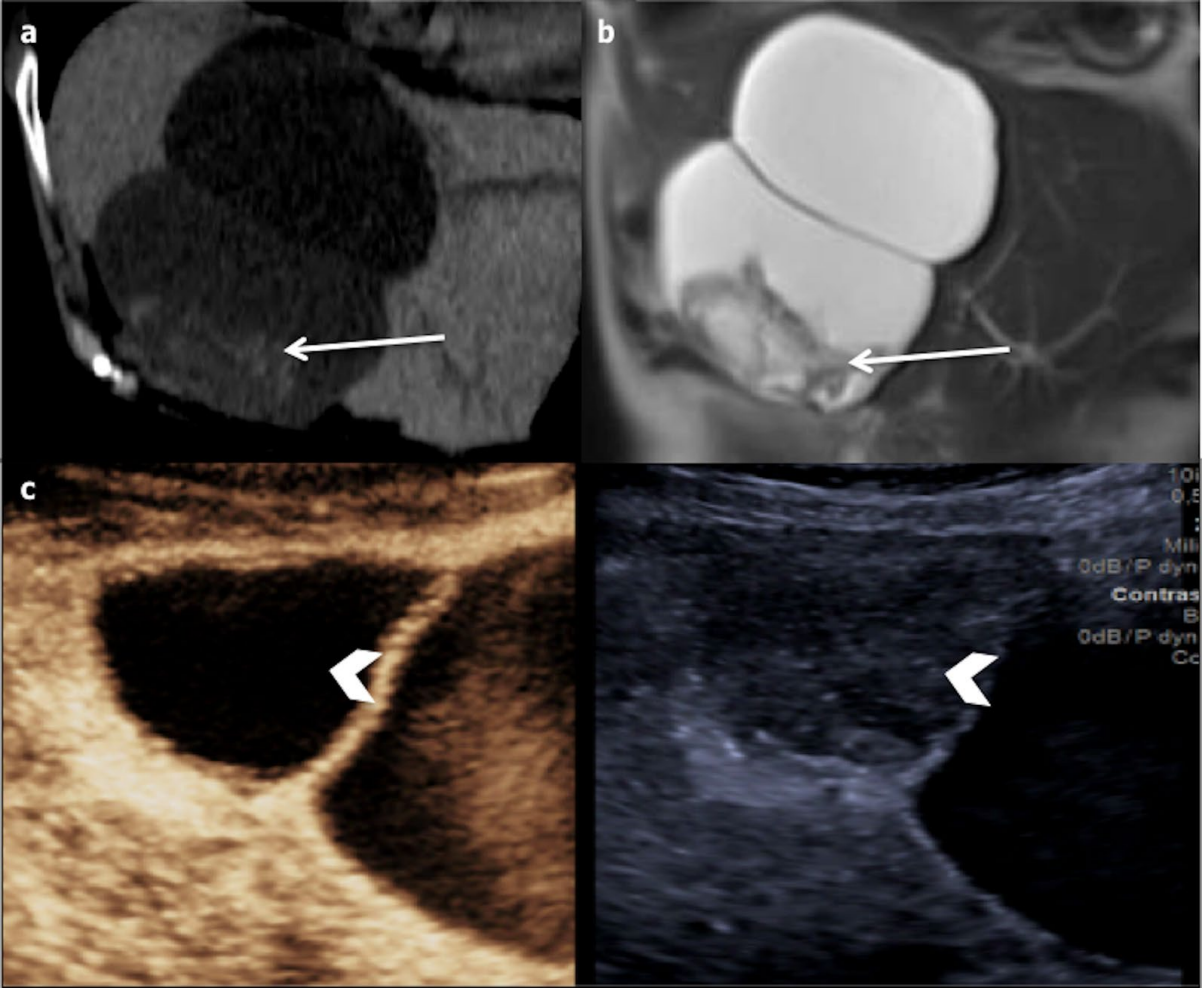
Bilobulated haemorrhagic hepatic cyst in a 73-year-old female. a Coronal non-enhanced computed tomography shows a bilobulated cyst with focal hyperattenuation in the inferior part (arrow); b coronal T2-weighted magnetic resonance imaging shows heterogeneous hypointensity inside the inferior part of the cyst (arrow). c Contrast-enhanced ultrasonography confirms the non-enhancement of the cystic part of the lesion (arrowhead)
